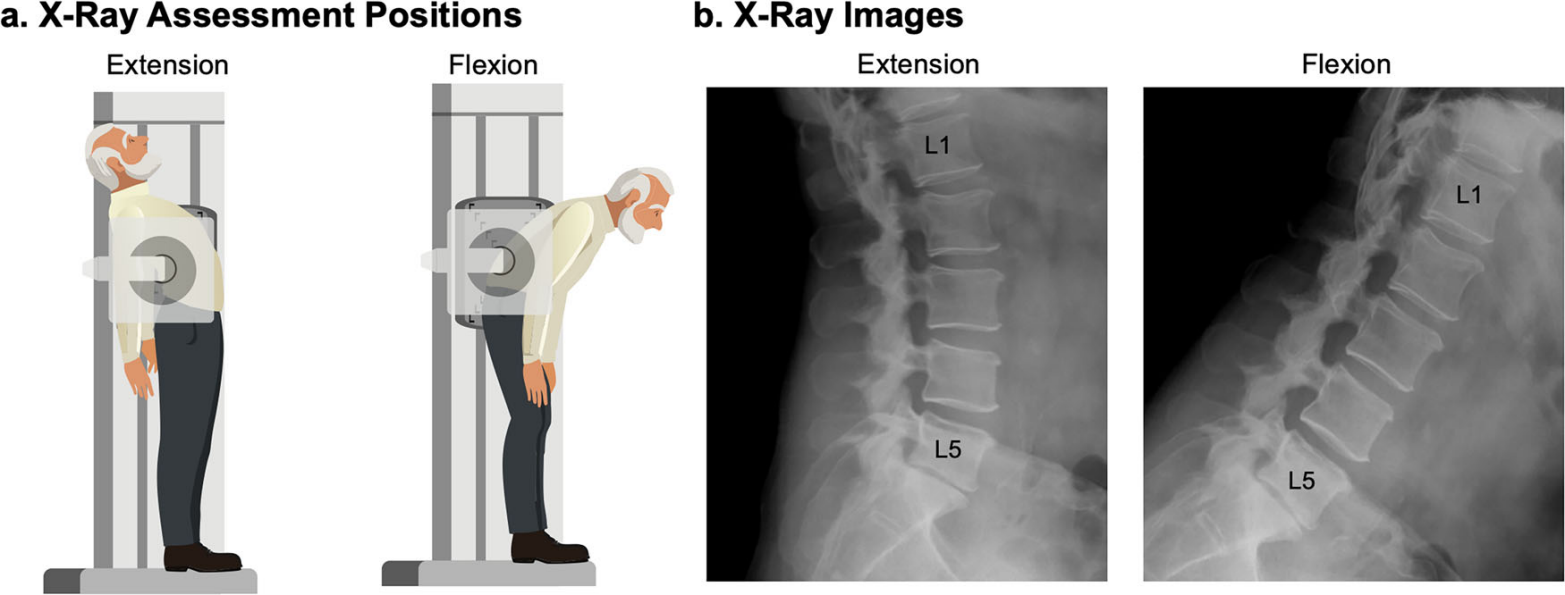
Radiography. Disc mechanics can be indirectly assessed from repeated radiographs under a postural loading, for example extension and flexion. Disc boundaries are measured by their adjacent bony locations to calculate change in disc height, wedge angle, or translation. b Lumbar spine extension and flexion X-ray images.
